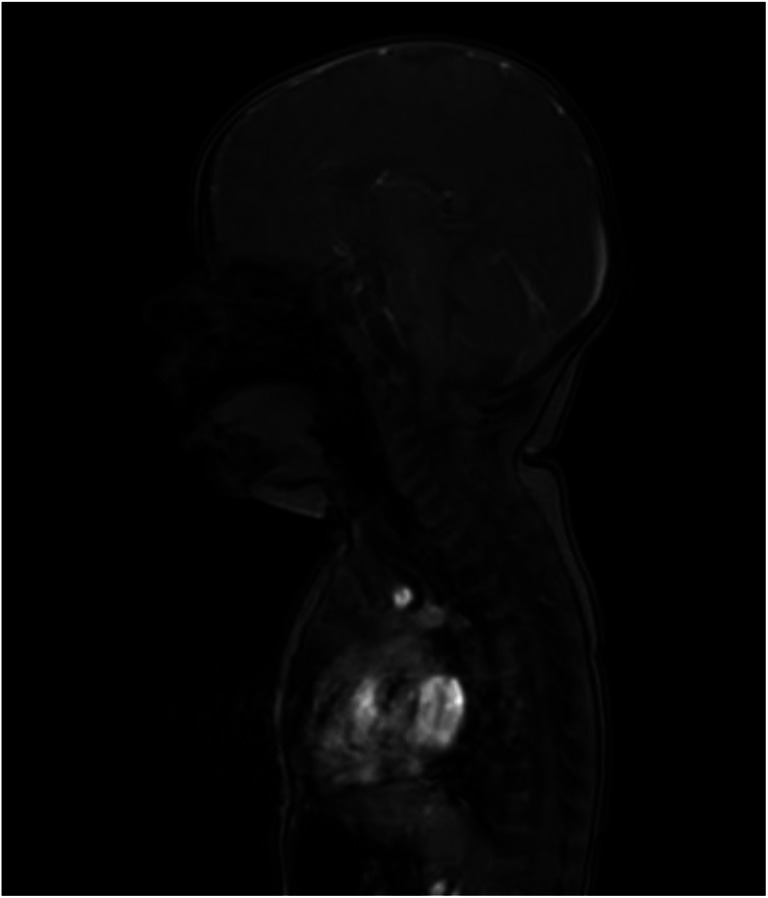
MRI of patient with a tumor adjacent to left subclavian artery
